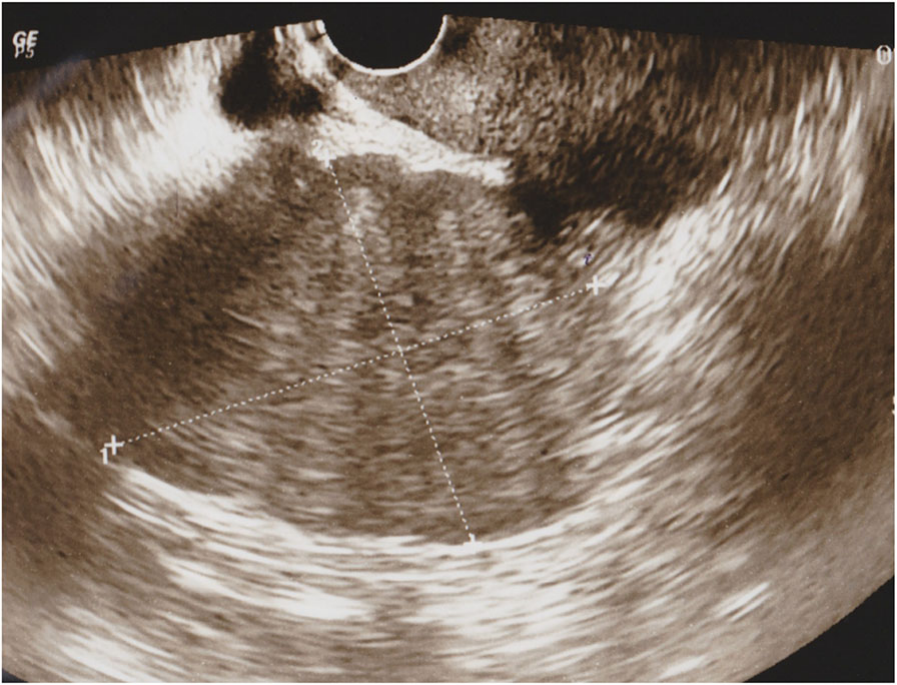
Transvaginal ultrasonography. A solid tumor margin measuring 10 × 8 cm in size is visible in the cranial part of the uterus
